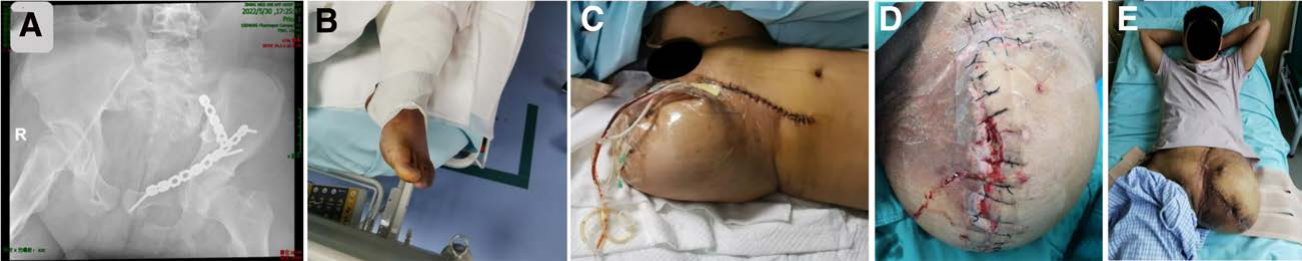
(A) Postoperative X-ray showing good alignment. (B) Poor postoperative peripheral blood flow. (C) After amputation. (D) Infection at the site of trauma after amputation. (E) Stump healing after amputation.
